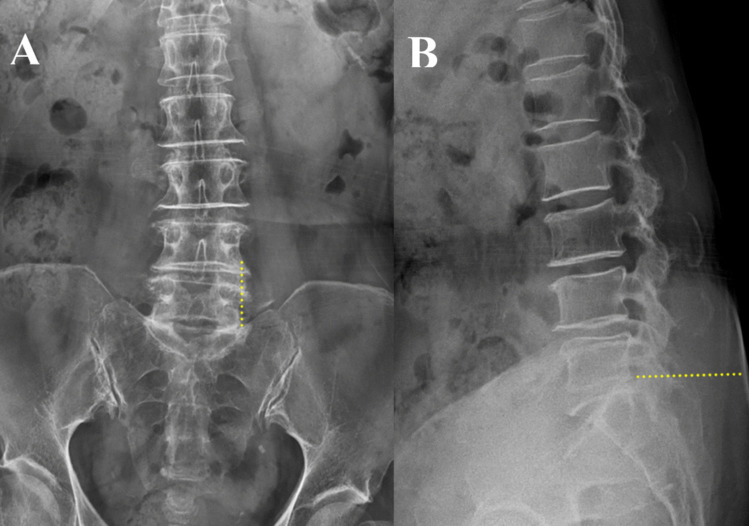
AP and lateral view of x-ray images. A AP view where the yellow dotted line represents the skin incision line aligning with the lateral margins of the pedicles. The caudally prominent tip of the left L5 TP, identified on the oblique coronal MR image, is also visible on the AP X-ray. B In the lateral view, the skin incision should be made at the right end of the dotted line, oriented toward the posteroinferior corner of the L5 vertebral body
